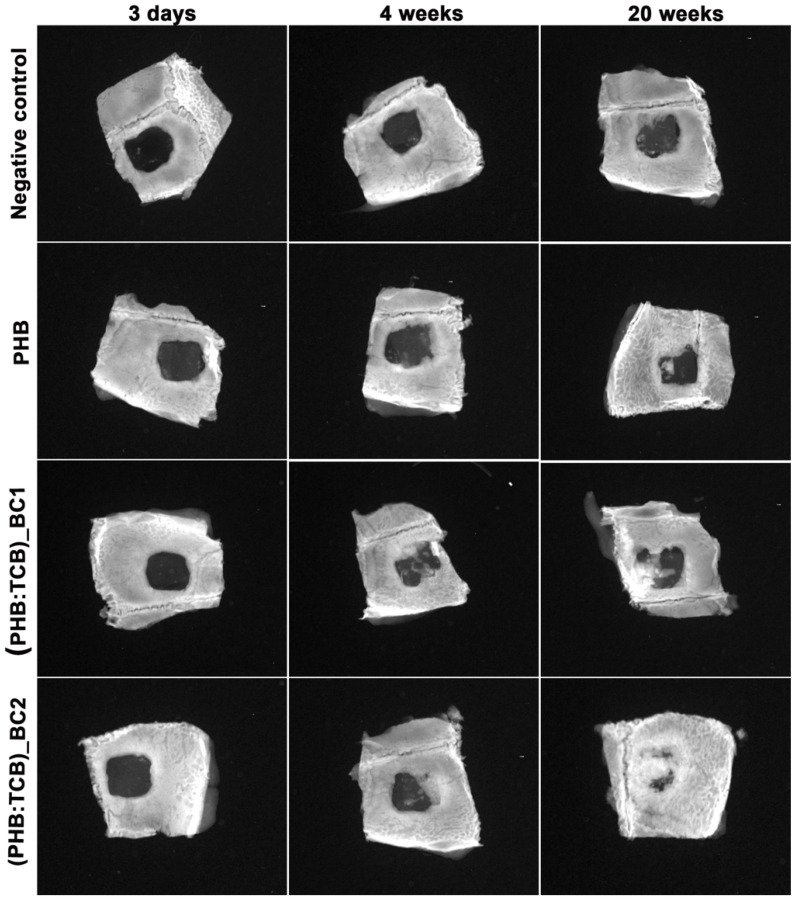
Radiographs of the in vivo bone samples taken after 3 days, 4 and 20 weeks of BC/PHB scaffold implantation. Radiological analysis showing gradual increase in bone formation in BC/PHB matrix compared to PHB matrix. Magnification ×2.5.
